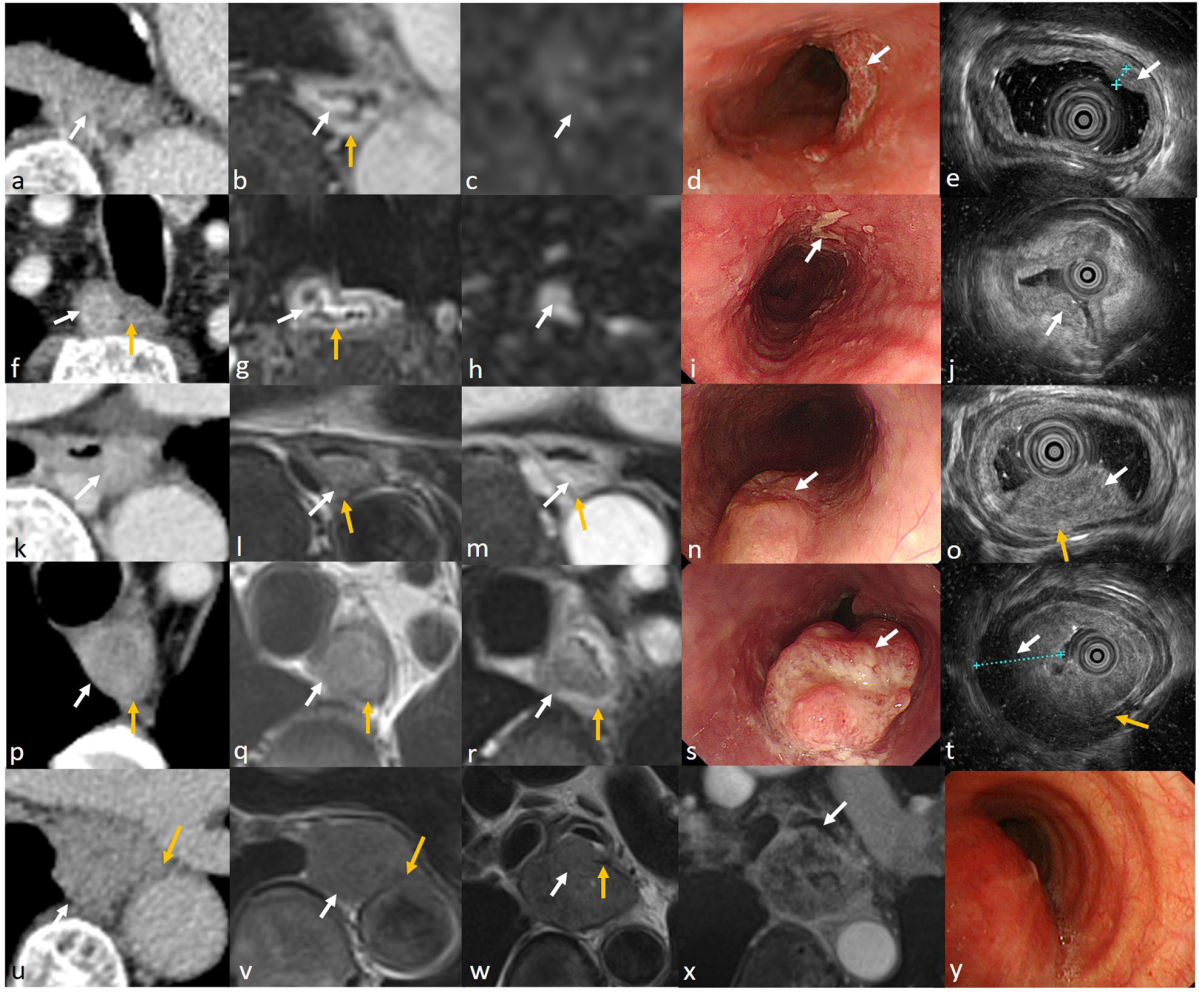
Stage T1-4 esophageal cancer on MR imaging. Stage T1a (a–e), it is difficult to detect the lesion on contrast-enhanced CT image (a). The lesion shows an intensely enhancing homogenous pedunculated mass (white arrow), with a degree of enhancement similar to that of the mucosa (yellow arrow) after contrast injection on T1-weighted image (b) and no restricted diffusion (c). Stage T1b (f–j), the lesion shows heterogeneously enhancing mass (white arrow) on contrast-enhanced CT image (f), similar to normal mucosa (yellow arrow), tumor blurs intensely enhanced mucosa after contrast injection on T1-weighted image (g) and has restricted diffusion (h). Stage T2 (k–o), it is difficult to detect the lesion on contrast-enhanced CT image (k). The tumor (white arrow) invades muscularis propria (yellow arrow), which is blurred on the T2-weighted image (l) and on the contrast-enhanced T1-weighted image (m). Stage T3 (p–t), the lesion (white arrow) appears to invade muscularis propria (yellow arrow) on contrast-enhanced CT image (p), however, the tumor involves muscularis propria and invades adjacent fat and fibrosis tissue. The muscularis propria is interrupted on the T2-weighted image (q) and on the contrast-enhanced T1-weighted image (r). Stage T4 (u–x) in two cases, the tumor (white arrow) appears to invade the aorta (yellow arrow) on contrast-enhanced CT image (u). The tumor invades the aorta on the T2-weighted image (v). The hypointense adventitia of the left principal bronchus (yellow arrow) is interrupted on the T2-weighted image (w) and on the contrast-enhanced T1-weighted image (x). The lesion is shown in the oesophagoscope (d, I, n, and s) and endoscopic ultrasonography (e, j, o, and d). The bronchoscope(y) shows the lesion invades the outer membrane of the left principal bronchus, while the inner membrane is intact
